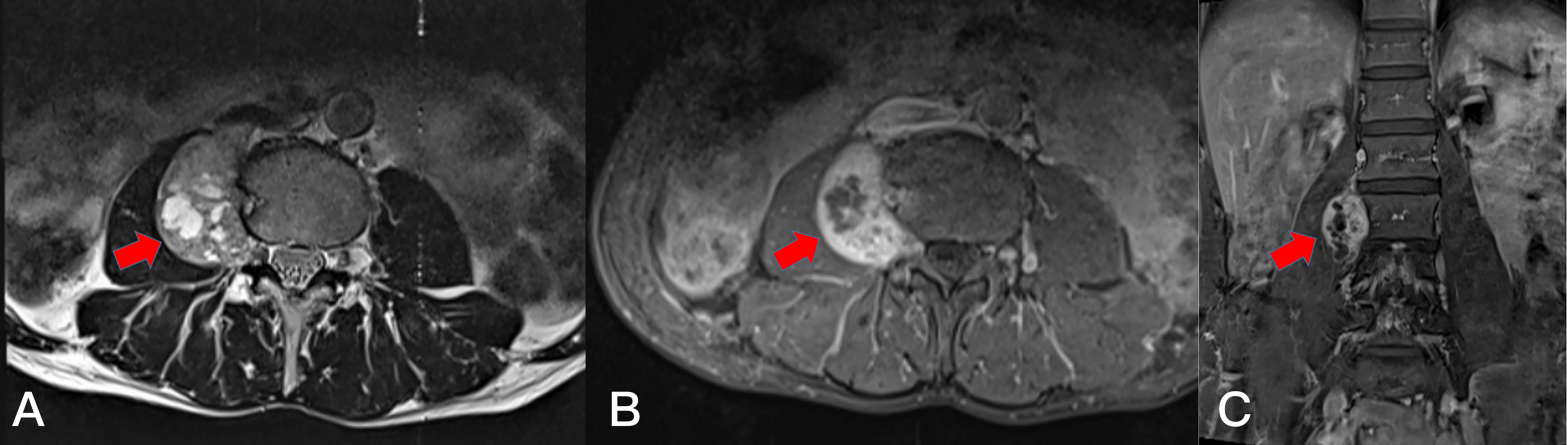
Preoperative T2-weighted MRI on axial (A) plane, T1-weighted enhanced MRI on axial (B) plane and sagittal (C) plane, revealing a dumbbell tumor (Eden IV) in the right L3-4 segment (red arrows).
